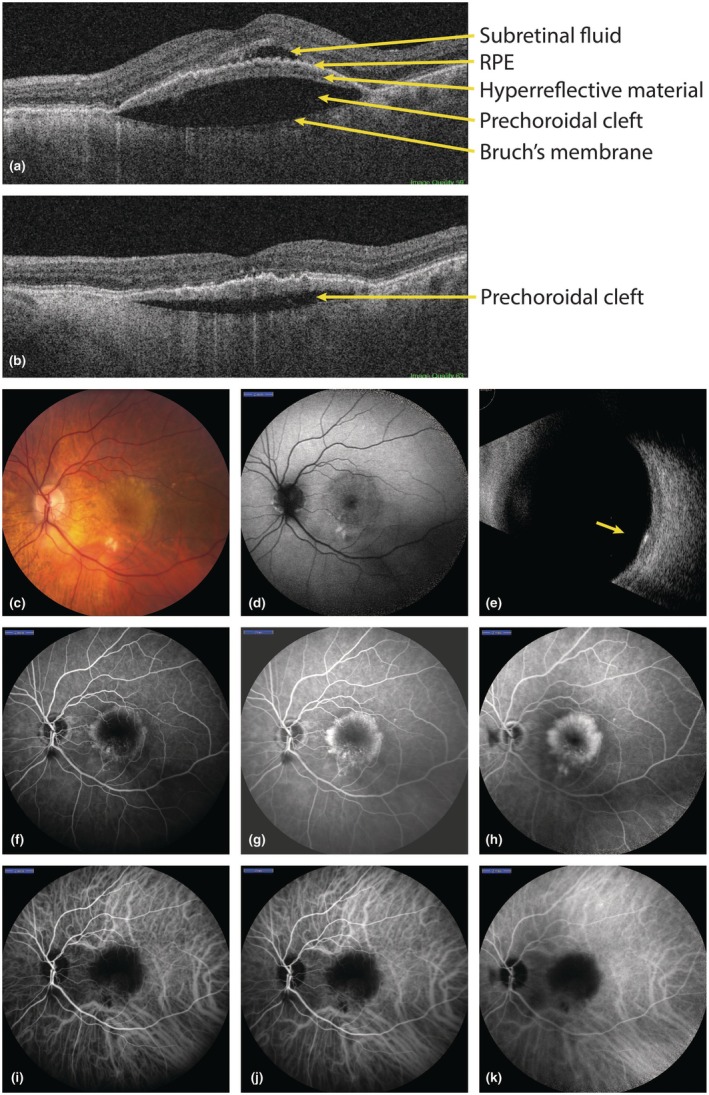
Clinical presentation of a large prechoroidal cleft in a 80‐year‐old patient who was under anti‐vascular endothelial growth factor (VEGF) therapy for neovascular age‐related macular degeneration (nAMD). (a) The prechoroidal cleft is located between Bruch's membrane and a band of hyperreflective material underneath the retinal pigment epithelium (RPE). Note the subretinal fluid accompanying this case, indicative of active disease. (b) Same patient, 5 months later after treatment with anti‐VEGF injections and resolution of the subretinal fluid, and regression of the prechoroidal cleft. (c) Fundoscopy demonstrating the nAMD in this patient at the time of image A, with an extensive amount of drusen. (d) Fundus autofluorescence demonstrating a hypo‐autofluorescent fovea. (e) Ultrasound imaging showing a mid‐reflective retinal lesion (arrow), and the absence of choroidal lesions or pathology. (f–h) Fluorescein angiography showing central leakage (image F at 1 min, G at 3 min, H at 10 min). (i–k) Indocyanine green angiography showing central blockage (image i at 1 min, j at 3 min, k at 10 min).
